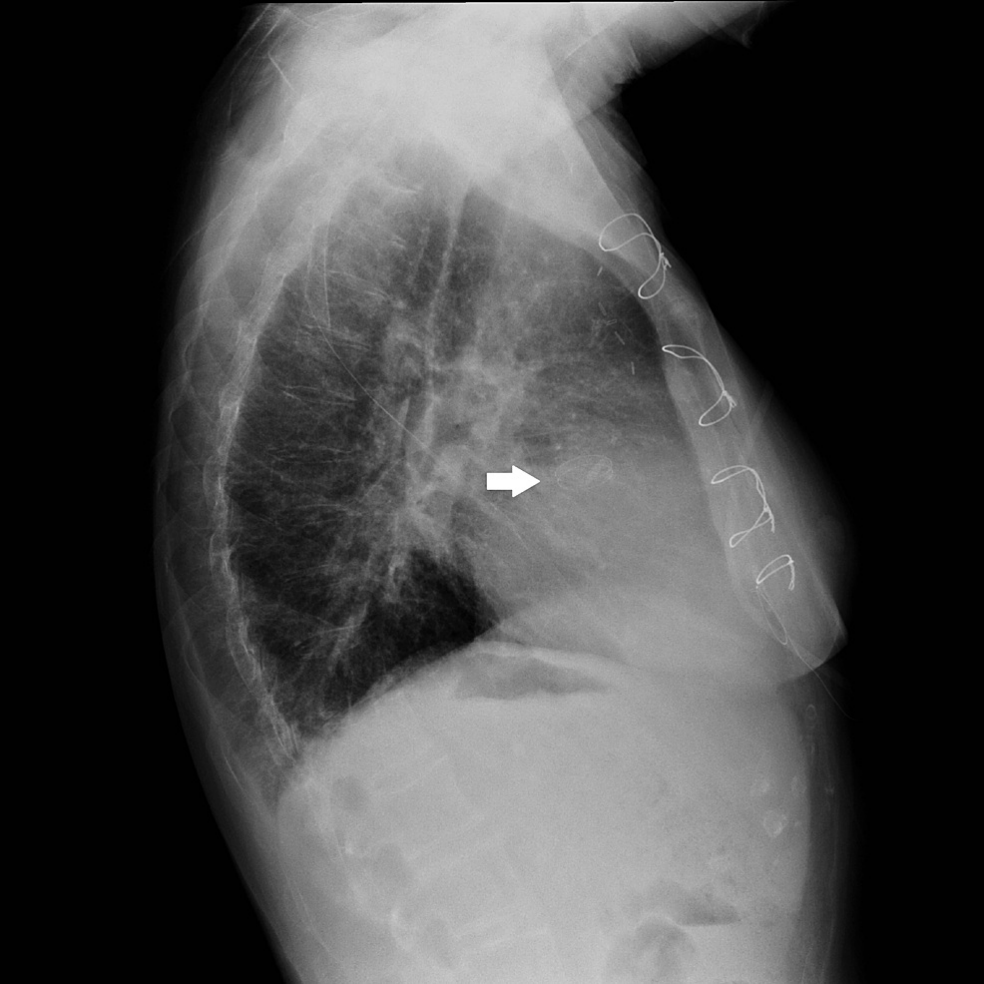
Lateral chest X-ray showing sternal wires, clear lungs, and a mechanical prosthesis projecting over the aortic valve position (indicated by a white arrow).
